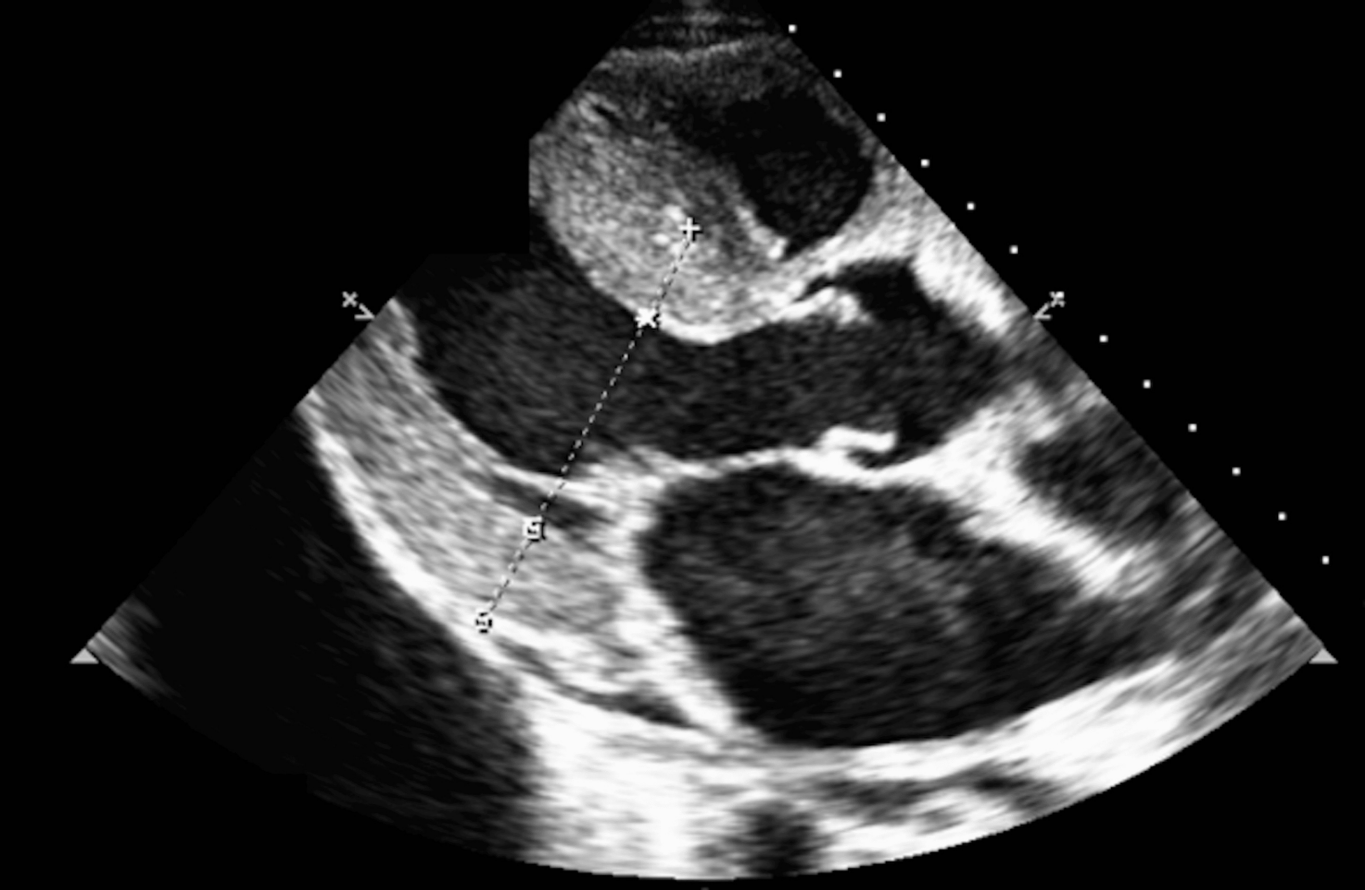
Parasternal long-axis echocardiographic view at the level of the papillary muscles Demonstrates marked left ventricular wall thickening (interventricular septum 1.61 cm; posterior wall 1.67 cm), consistent with an infiltrative cardiomyopathy. The left ventricular cavity appears small.
